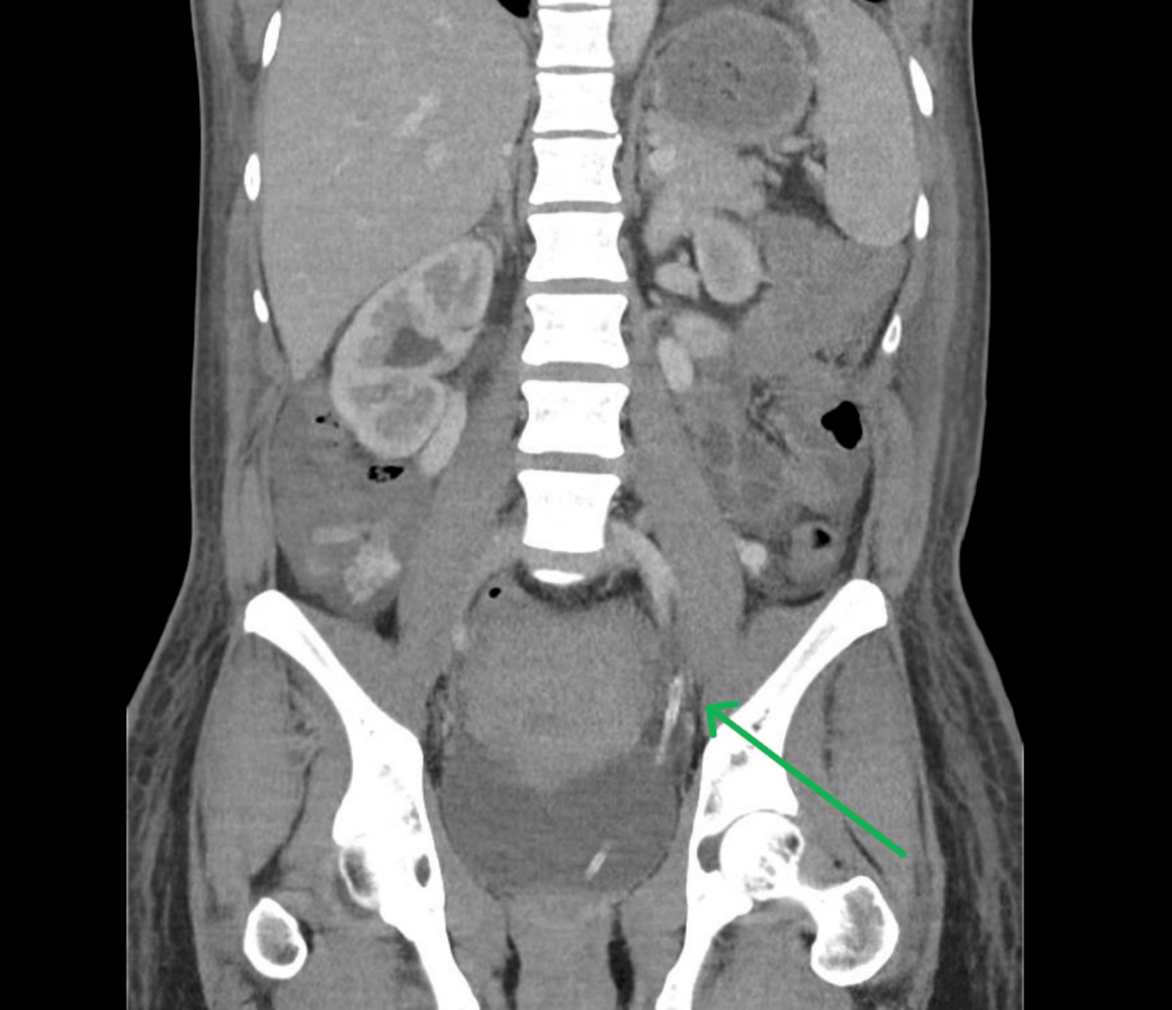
Computed tomography (CT) coronal
